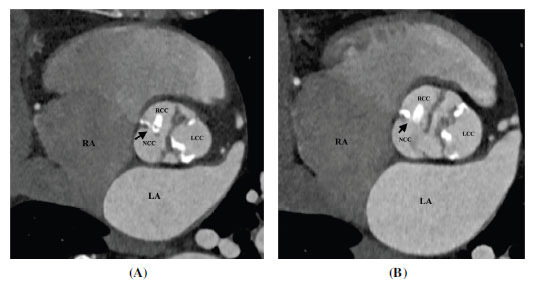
Type 1 (R-N) bicuspid aortic valve. (A) Axial contrast-enhanced computed tomographic angiography image diastolic frame and (B) Axial contrast-enhanced computed tomographic angiography image systolic frame image showing bicuspid aortic valve with a thick calcified raphe between right and non-coronary cusps (R-N subtype). Abbreviations: RA; right atrium, RVOT; right ventricular outflow tract, LA; left atrium, LV; left ventricle, Ao; ascending aorta, RCC; right coronary cusp, LCC; left coronary cusp, NCC; non-coronary cusp.
